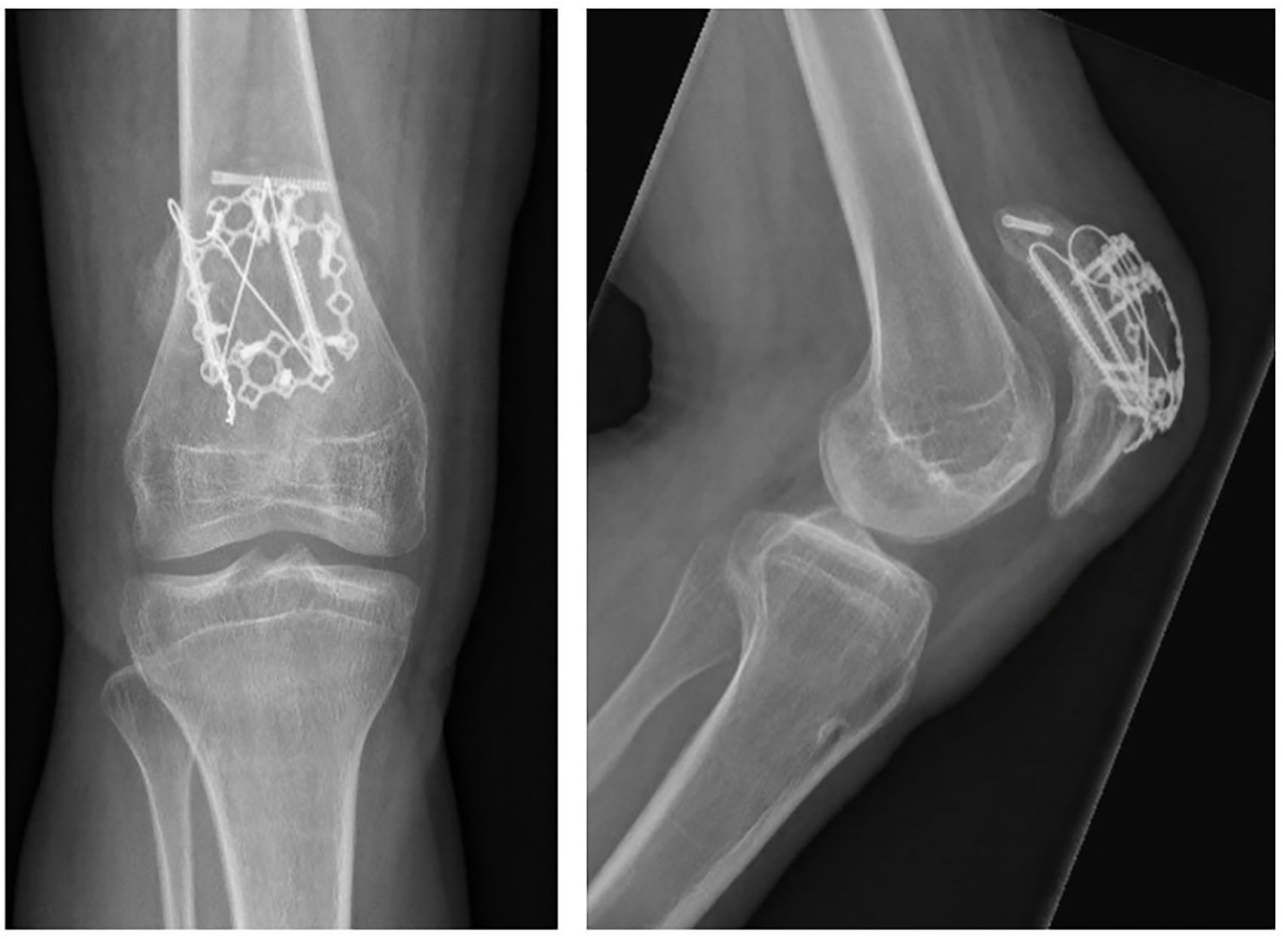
Plain AP and lateral radiographs at 3 months follow-up showing a healed patella.
